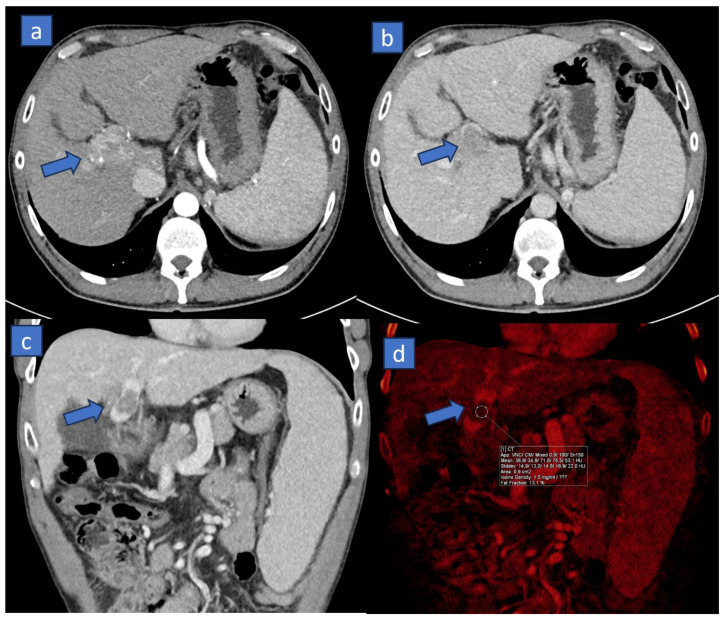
Sixty-five-year-old man suffering from liver cirrhosis with solid neoplastic thrombus of the portal vein. On the axial CT scan acquired during arterial phase, (a) a hypervascular liver tumor is depicted on hilum region, consistent with the diagnosis of hepatocellular carcinoma (arrow). On the corresponding venous-phase scan, (b) the lesion shows the typical wash-out pattern (arrow). On the coronal CT reconstruction, (c) a hypodense filling defect is recognized inside the portal vein (arrow). On the corresponding DECT reconstruction, (d) the assessment of DECT numbers (mean density: 36.9 HU; iodine density: 1.5 mg/mL) calculated from iodine map shows a clear enhancement of the solid neoplastic thrombus (arrow).
